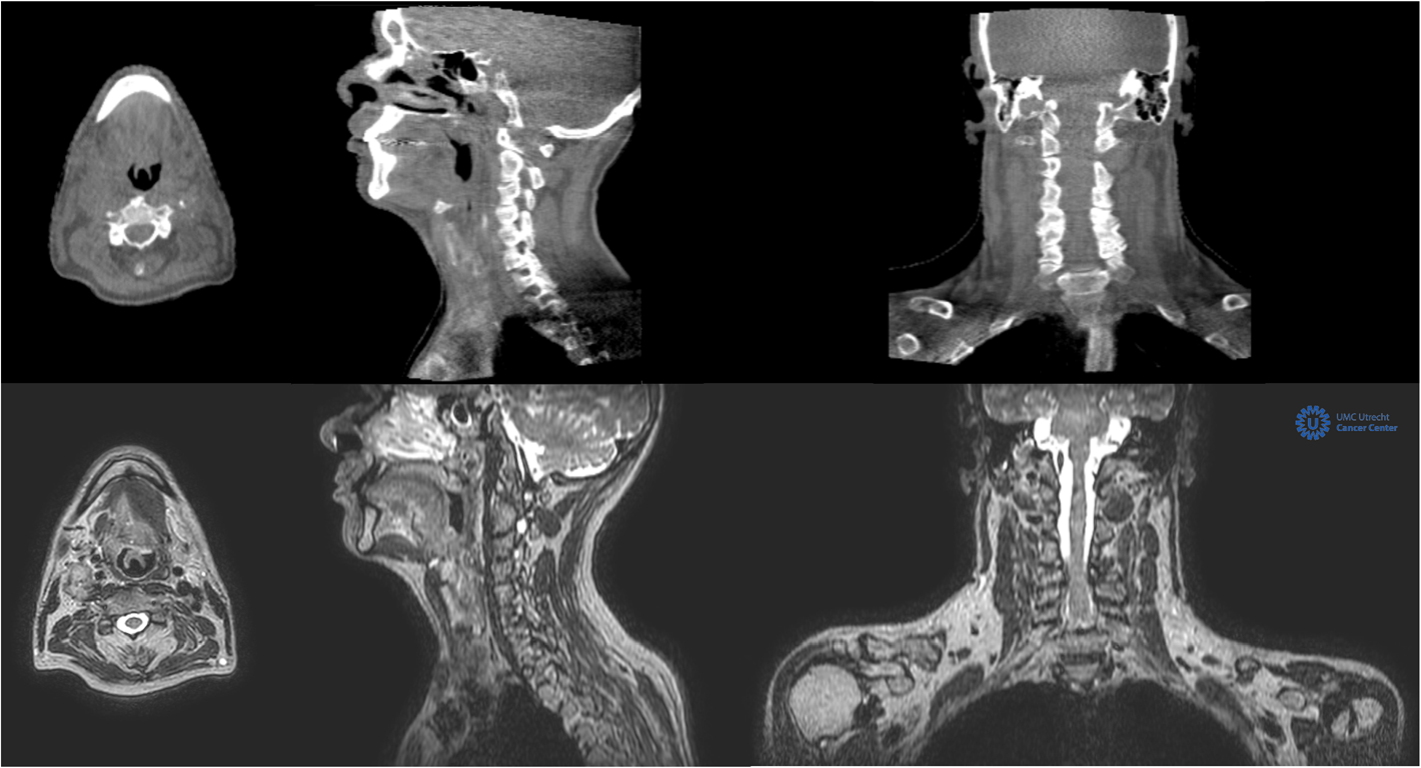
Cone beam CT images of an oropharyngeal cancer patient (upper row) compared to the 1.5 T MR images (T1 3D 0.7 × 0.7 × 1.2 mm^3^) of the same patient acquired at the MRL (lower row)
